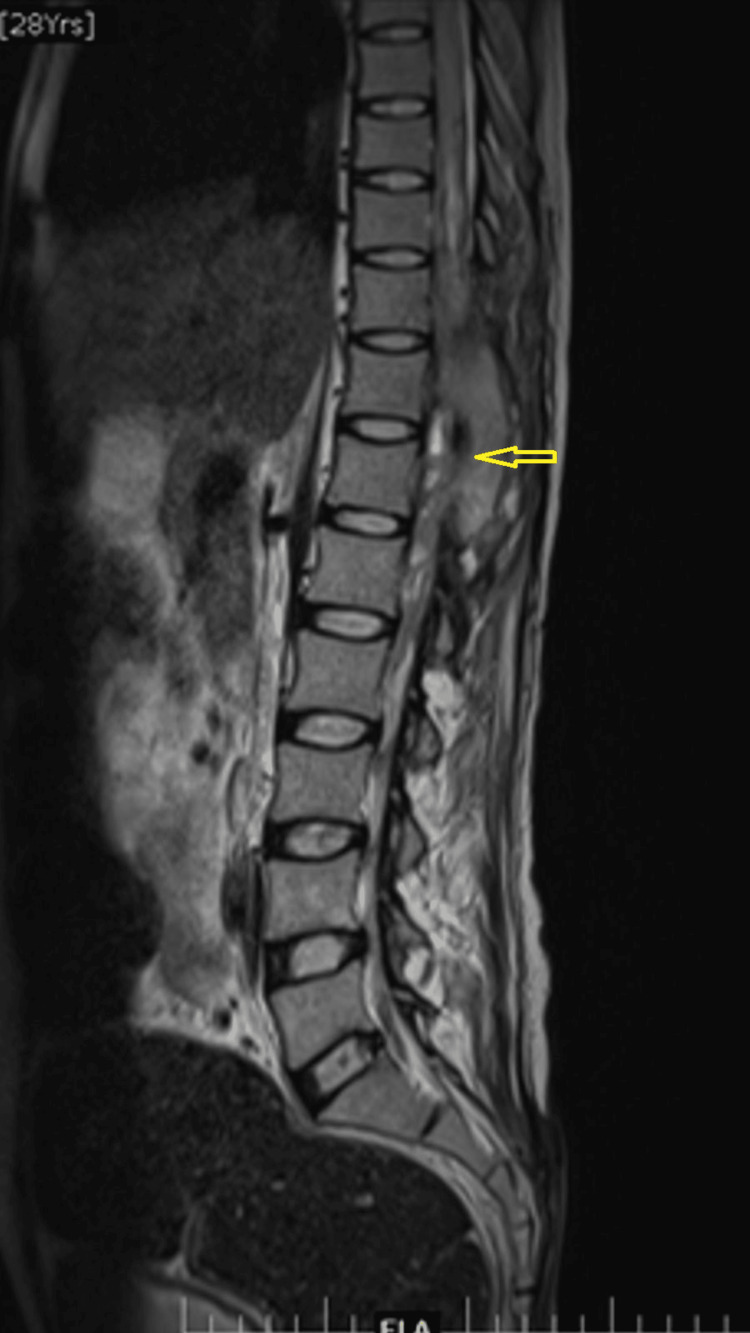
An MRI of the thoracolumbar spine after six weeks of treatment showed a decrease in cord edema, resolution in collection, and herniation of the cord (yellow arrow).
